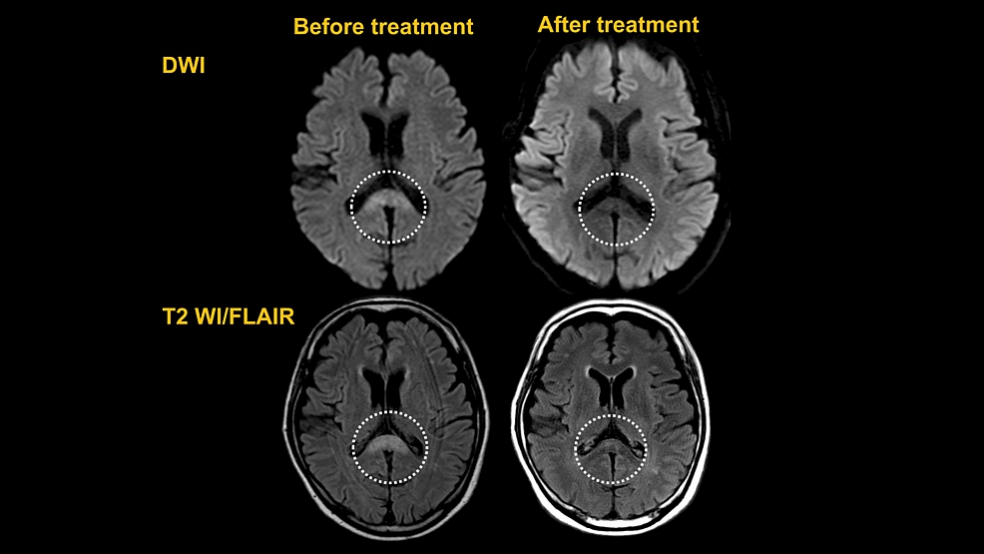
Head MRI (DWI and T2/WI FLAIR) Head MRI showing the changes that took place after treatment. The DWI and T2 WI/FLAIR signals show high density and swelling of the corpus callosum before treatment and atrophy and high-density improvement in the same area after treatment DWI: diffusion-weighted imaging; MRI: magnetic resonance imaging; T2 WI/FLAIR: T2-weighted/fluid-attenuated inversion recovery
